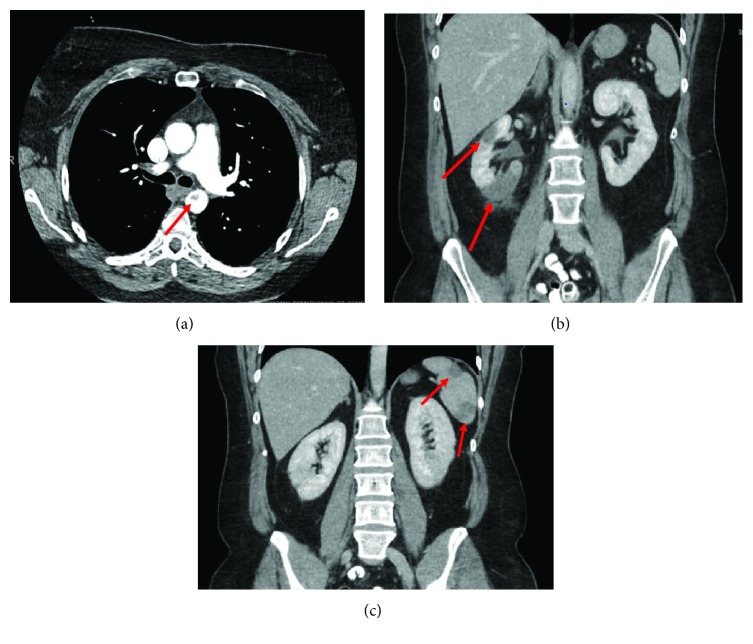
CT of the abdomen and pelvis revealing a mass in the descending thoracic aortia (a) with wedge-shaped infarctions in the right kidney (b) and spleen (c) consistent with an embolic phenomona.
